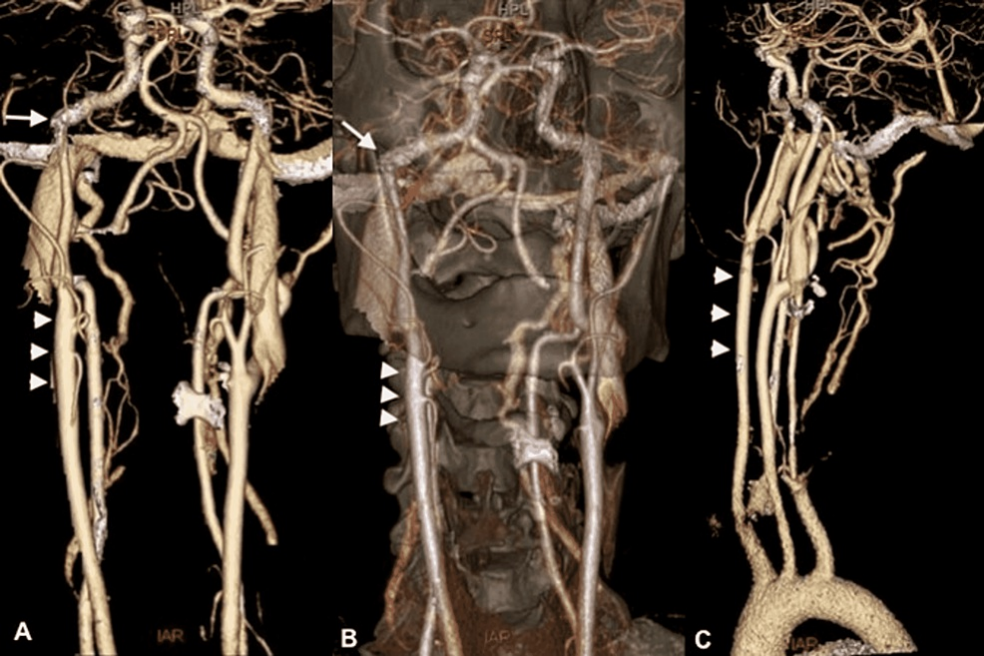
CTA neck shows a non-bifurcating carotid artery (NBCA) giving rise to the external carotid artery branches (A and B, arrow heads. The inferior branch: Superior Thyroid Artery. Two middle branches with close origin: Lingual and Facial Arteries. The superior branch: Superior Temporal Artery ), entering the skull base as the internal carotid artery (C, arrow heads), however with an additional variant, a lateralized carotid artery at the skull base (A, B, arrows).
